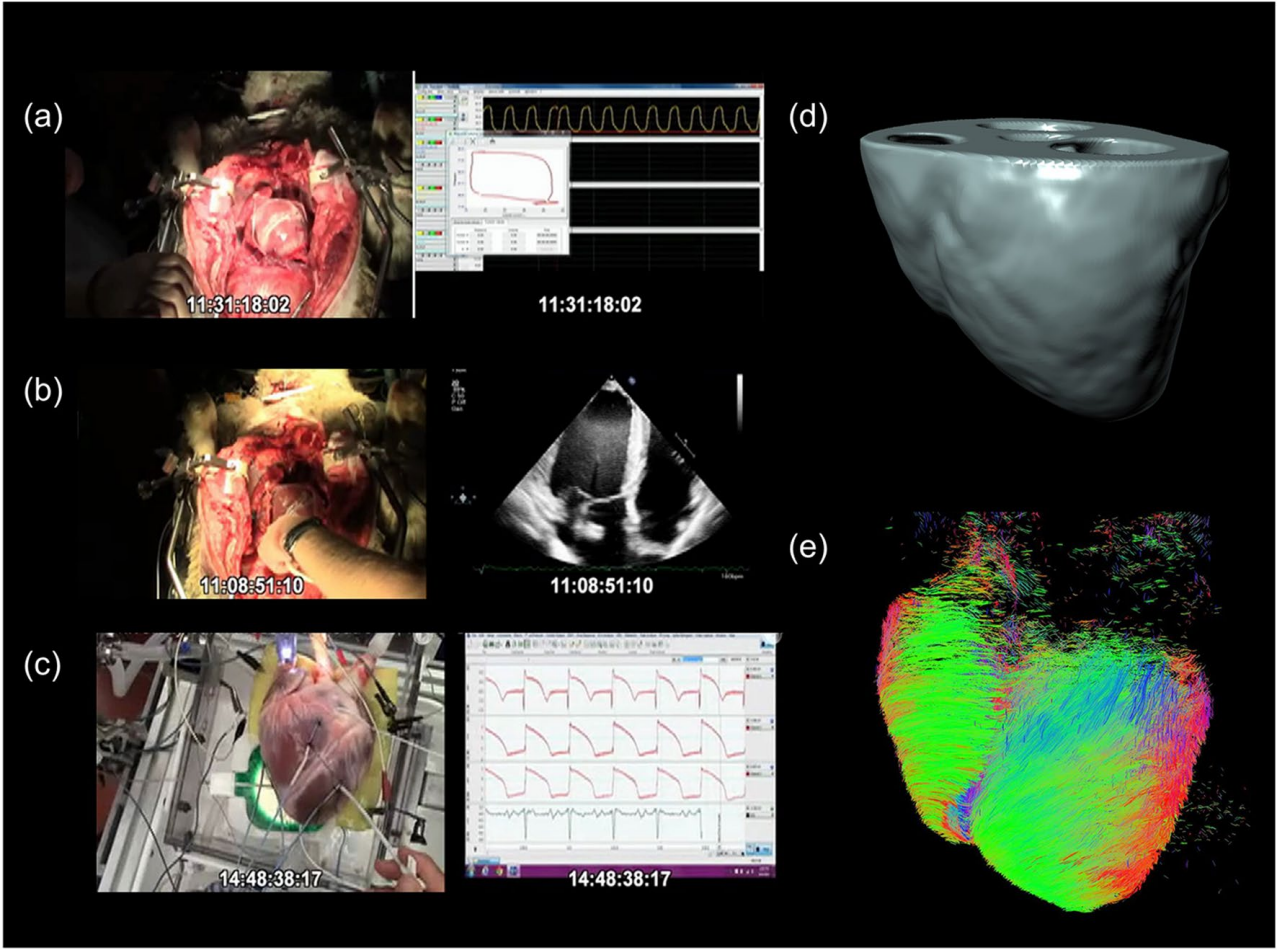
Data collection from a single heart for in silico model development. (a) Pressure volume loops, (b) echocardiography, and (c) epicardial monophasic action potentials were obtained in vivo and subsequently ex situ. (d) MRI and (e) DTMRI were employed to obtain accurate descriptions of anatomy (for FE mesh construction) and myofiber directions (to assign material heterogeneity and anisotropy).
